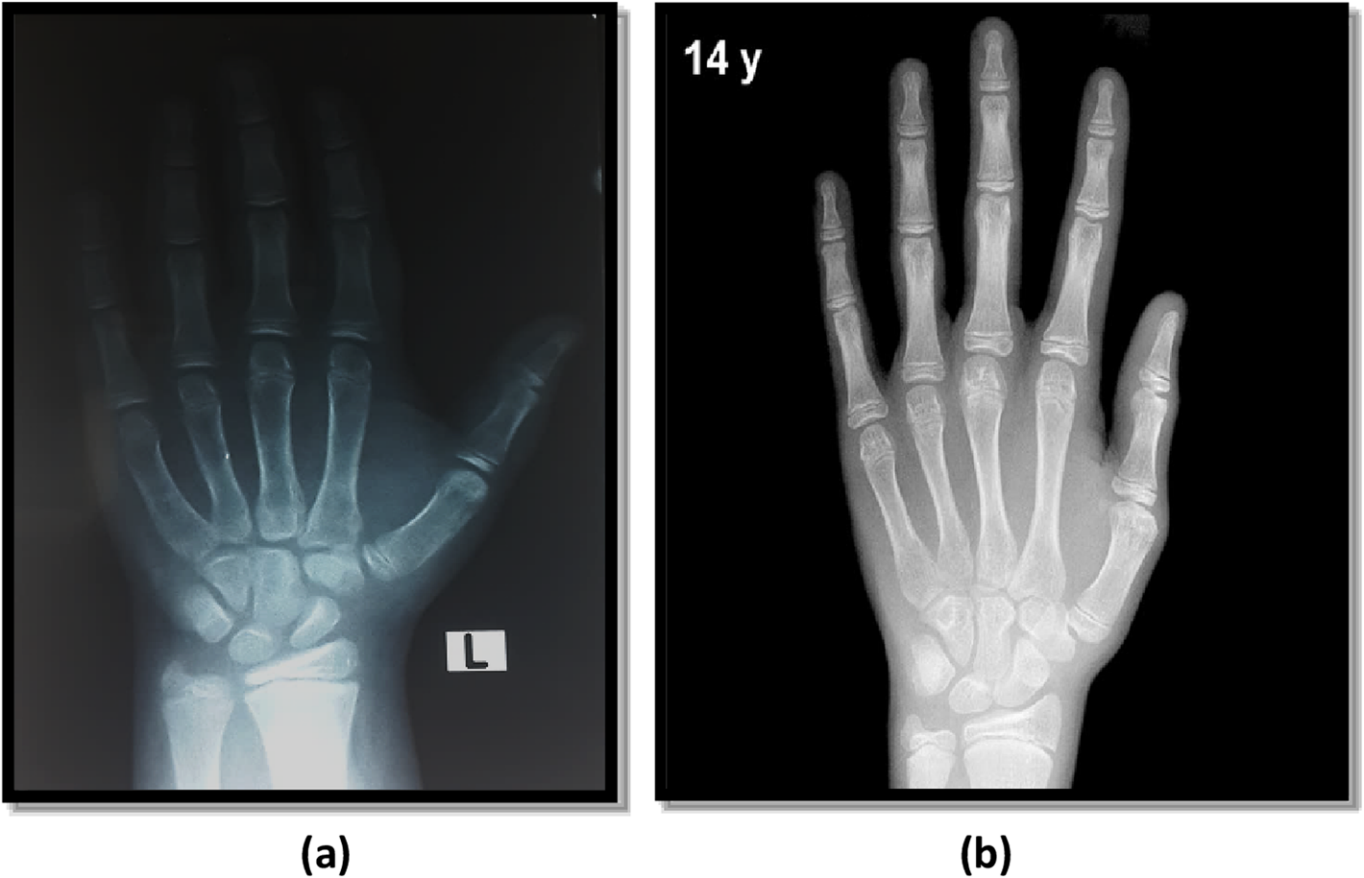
a Radiograph of the left hand obtained in a 12.3-year-old Egyptian boy. b Greulich and Pyle method atlas image showing advanced bone age (14 years old)
